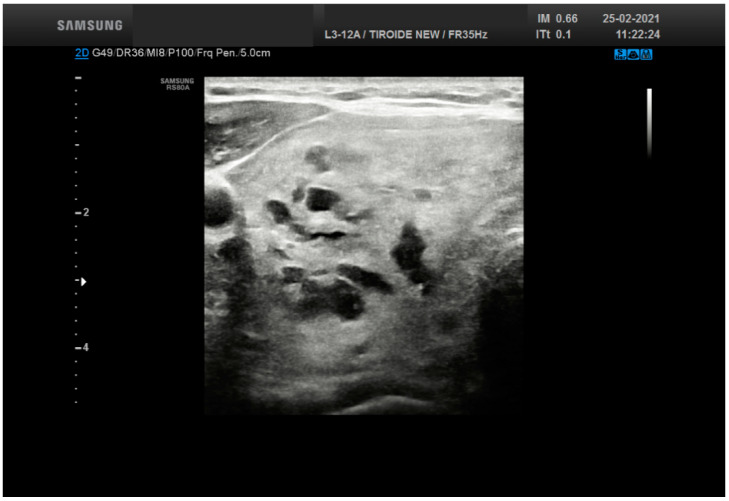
Ultrasound features from a thyroid nodule (50 mm size) resulting into a score 2 (solid and cystic plus hyperechoic-isoechoic) belonging to TR 2. The lesion was diagnosed as adenomatous goiter.
